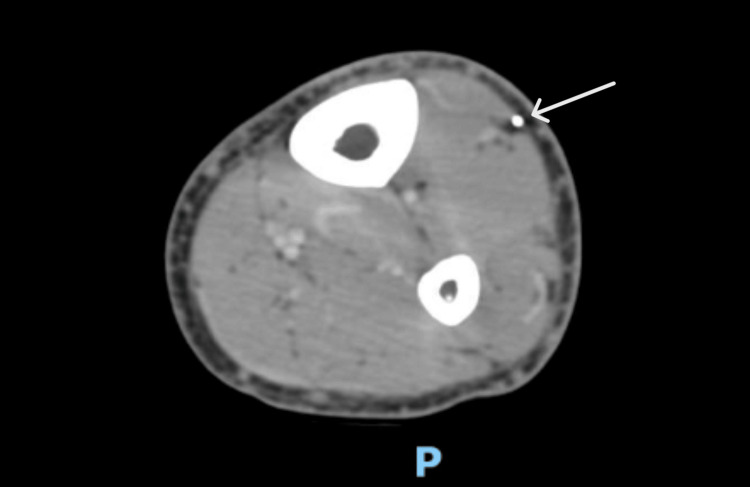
CT scan of the left lower extremity. Axial view of the image. The white arrow points toward the foreign body object. CT, computed tomography.
